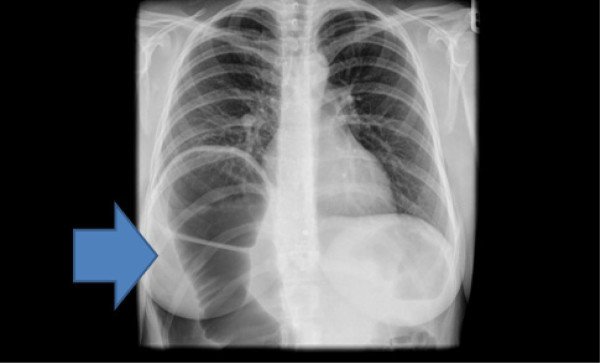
Radiograph of the chest showing gross dilatation of the right side of the colon with Chilaiditi’s sign.
